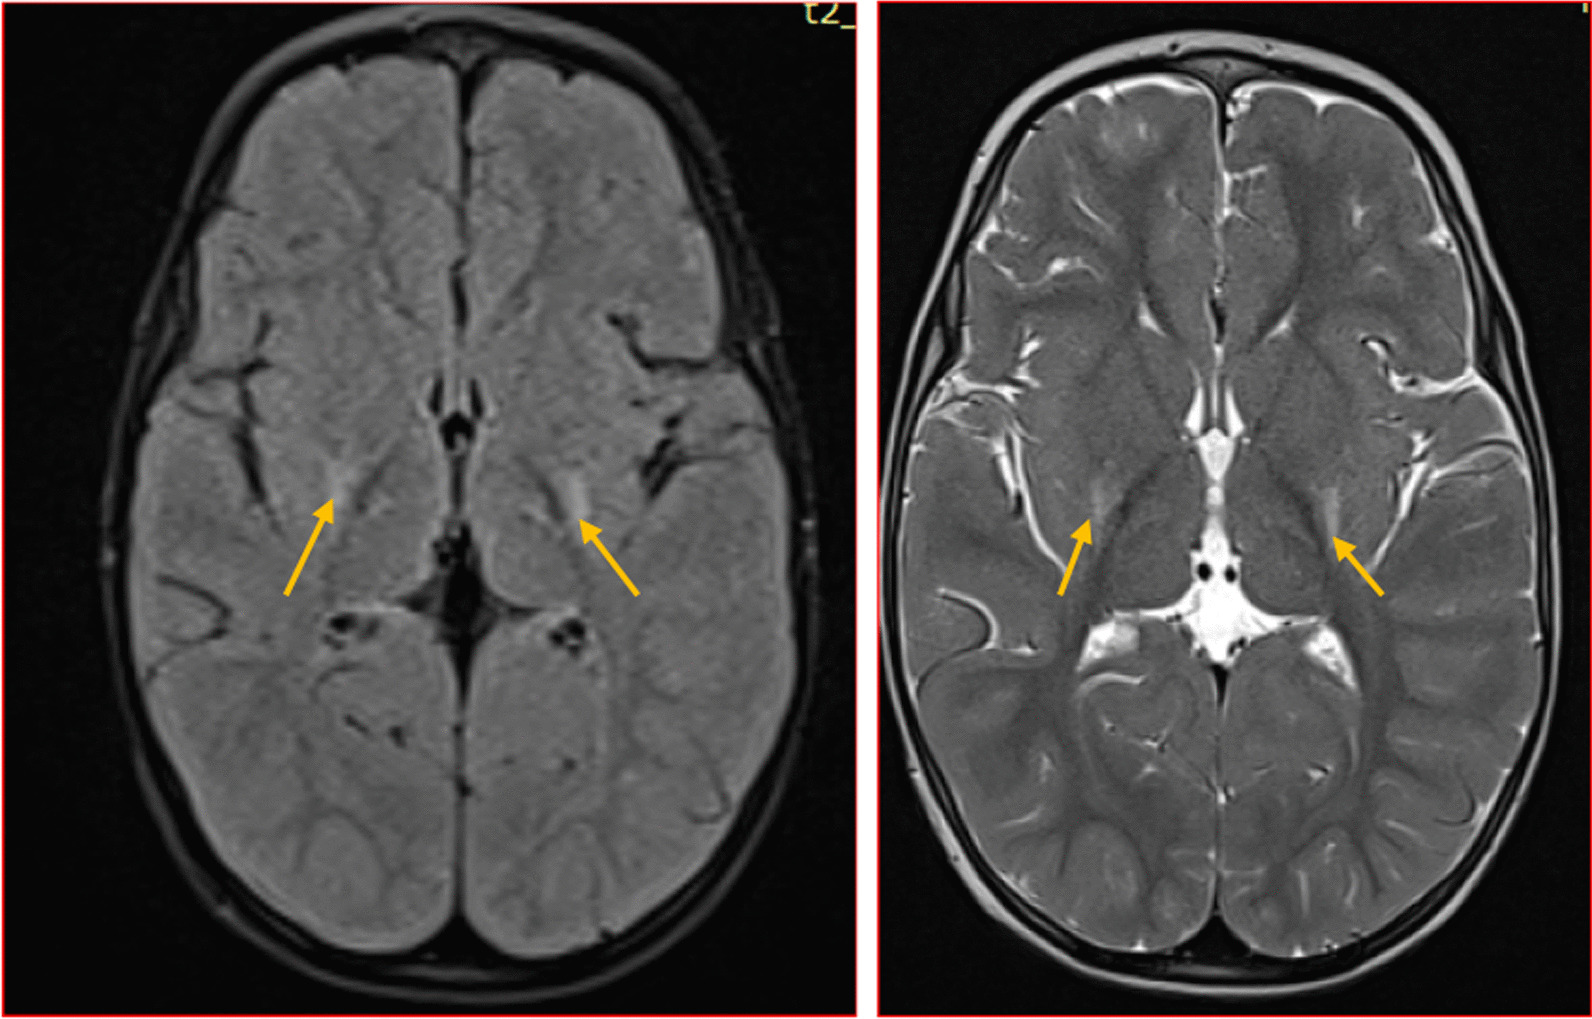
Two year old female with abnormal hand movements. Axial (T2/FLAIR) brain MRI demostrating symmetrical dorsal putaminal T2/FLAIR hyperintensities(arrows) highly suspicious for neuro-metabolic disorder
